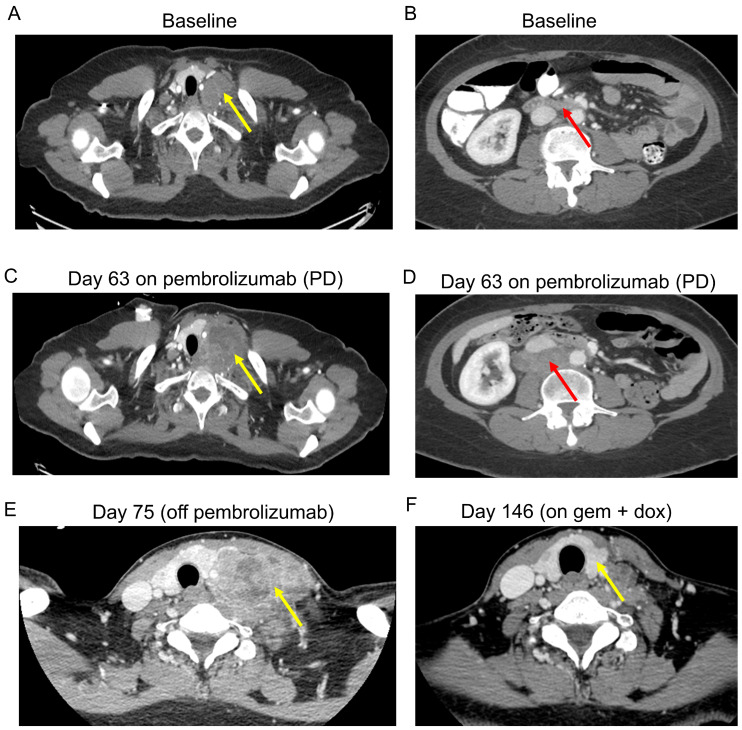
Patient 3 in the trial presented with gross hematuria and was found to have a 4.3 cm left renal mass with retroperitoneal adenopathy for which the patient underwent a left nephrectomy. Twenty days later, presented to MDACC with progressive disease in the retroperitoneum. Initiated on treatment with paclitaxel, carboplatin and bevacizumab and had disease progression after 5 cycles. Subsequently enrolled on a clinical trial with pembrolizumab. Panels (A,B) show disease burden in the mediastinum (yellow arrows) and retroperitoneal lymph nodes (red arrows) prior to initiation of pembrolizumab. The patient had radiographic (C–E) and symptomatic disease progression (PD) within 9 weeks from initiation of pembrolizumab and was urgently hospitalized 7 days after discontinuation for management of severe pain due a rapidly enlarging neck mass (E), back pain and syncopal episodes induced by the pressure of the mass against the carotid body. Emergently started on gemcitabine (gem) 900 mg/m^2^ over 90 min and doxorubicin (dox) 40 mg/m^2^ with rapid improvement in symptoms and radiographic response (F).
